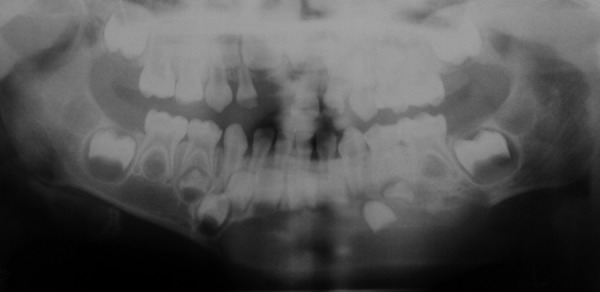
Postoperative panoramic radiograph showing bone formation at superoposterior aspect of 36 region
